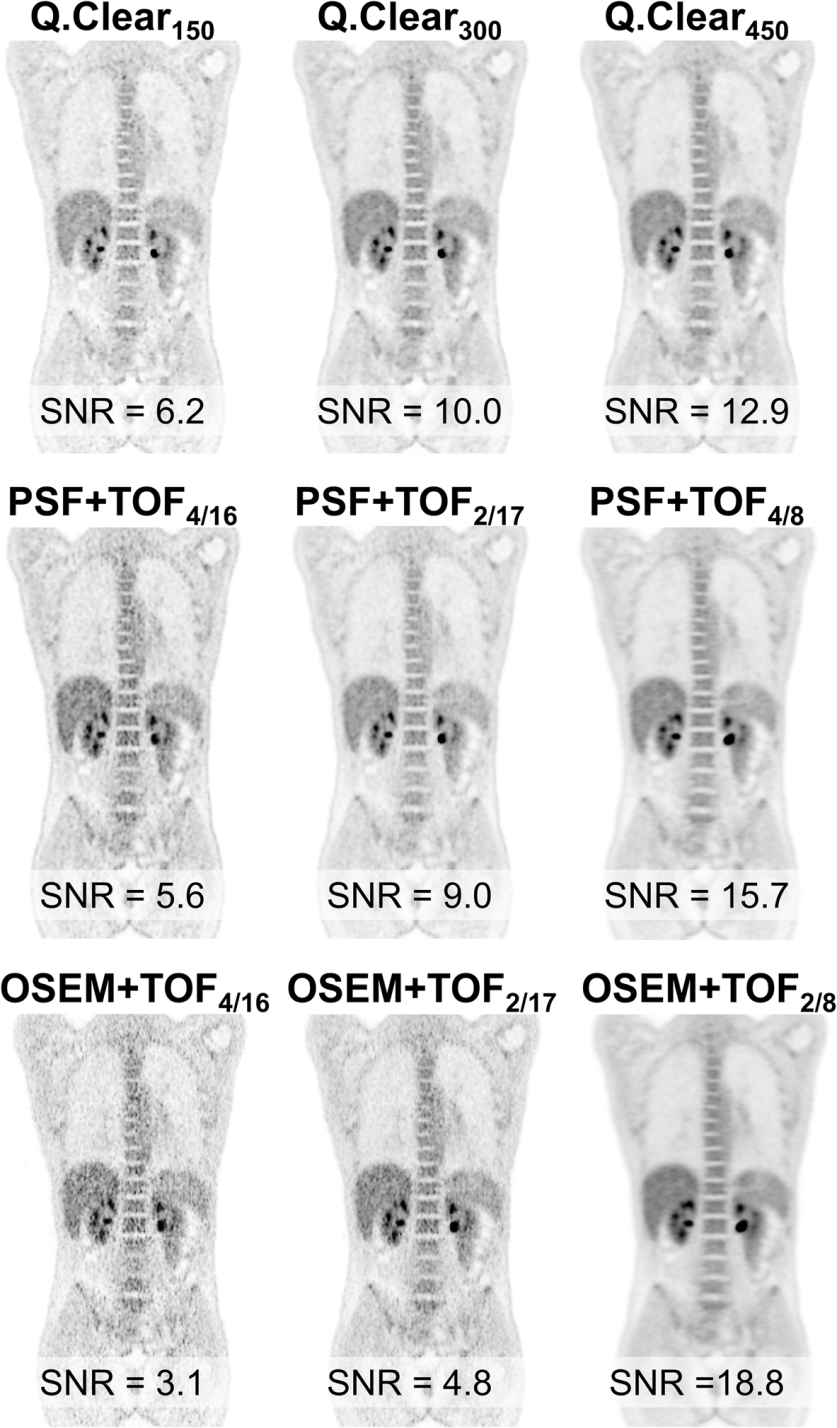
Patient example. Coronar FDG-PET images of a patient are displayed for all nine reconstruction algorithms (details see the “Methods” section). Signal-to-noise ratio (SNR) in the liver is given. Notably, Q.Clear_150_, PSF+TOF_4/16_, OSEM+TOF_4/16_, and OSEM+TOF_2/17_ exhibit unacceptably high noise (i.e., low SNR)
